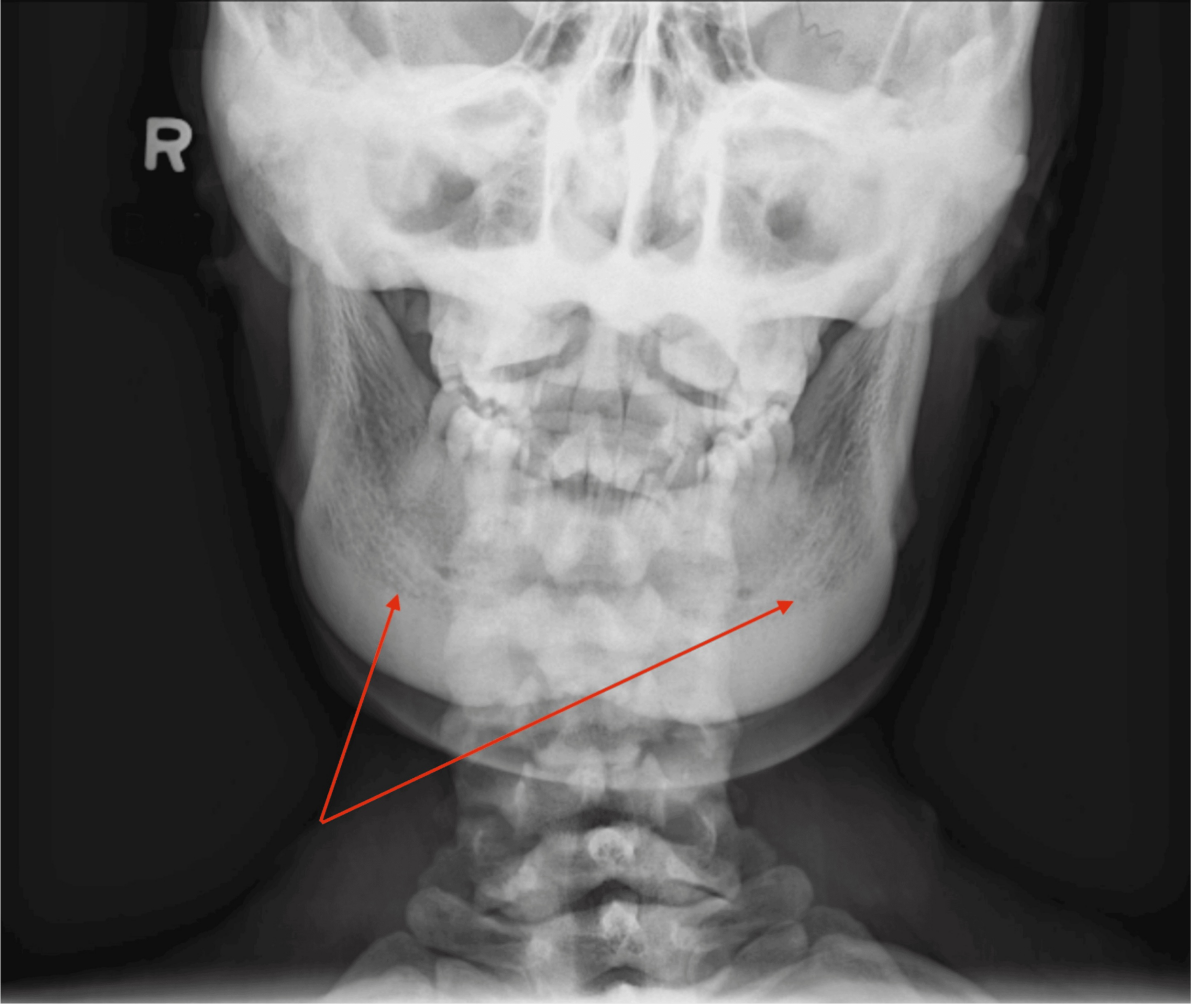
Posteroanterior X-ray of the mandible Diffusely increased bone mineral density with thickening of bilateral mandibular bodies
